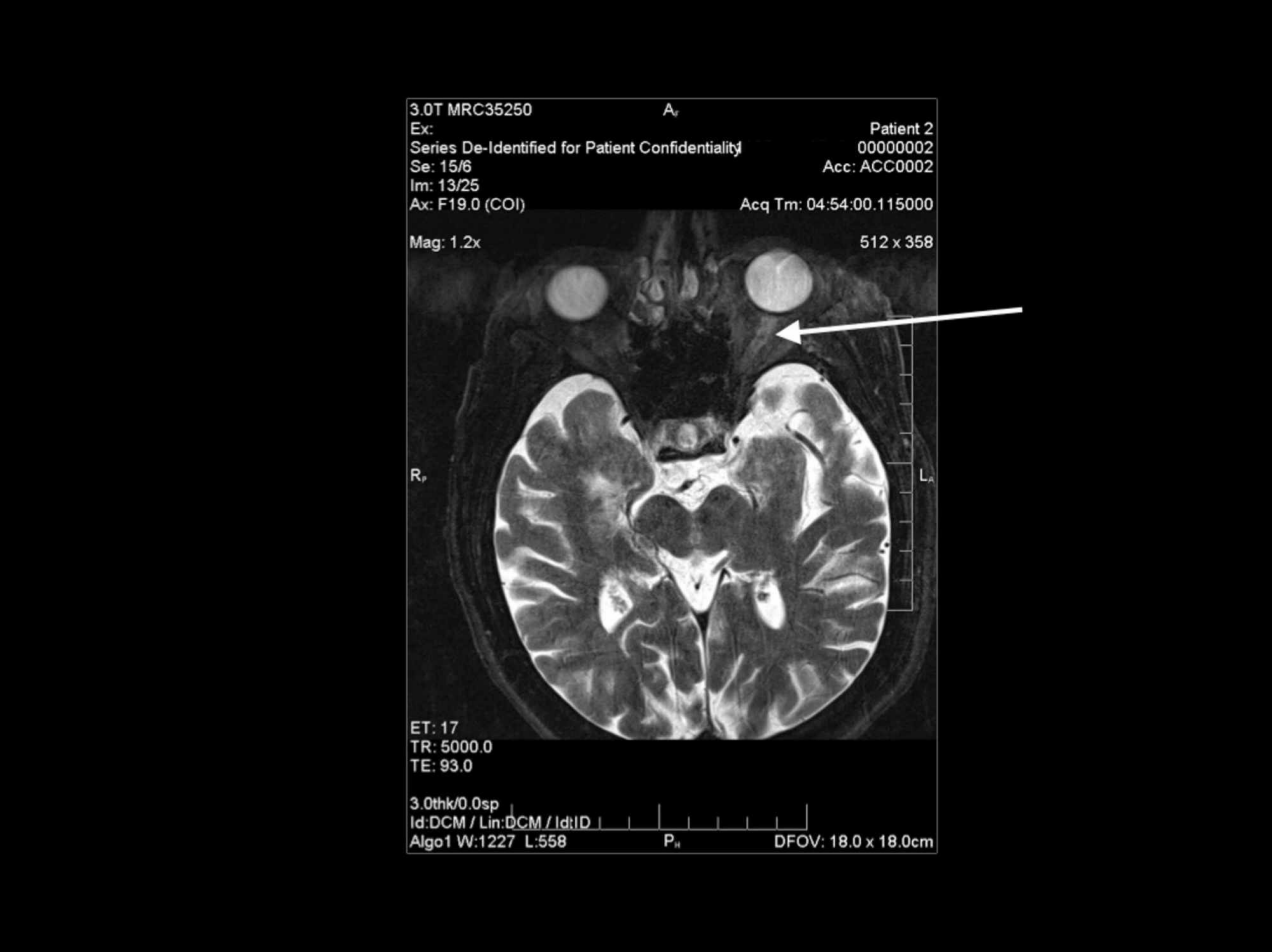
MRI showing perineural enhancement of the left optic nerve sheath (arrow) MRI: magnetic resonance imaging
